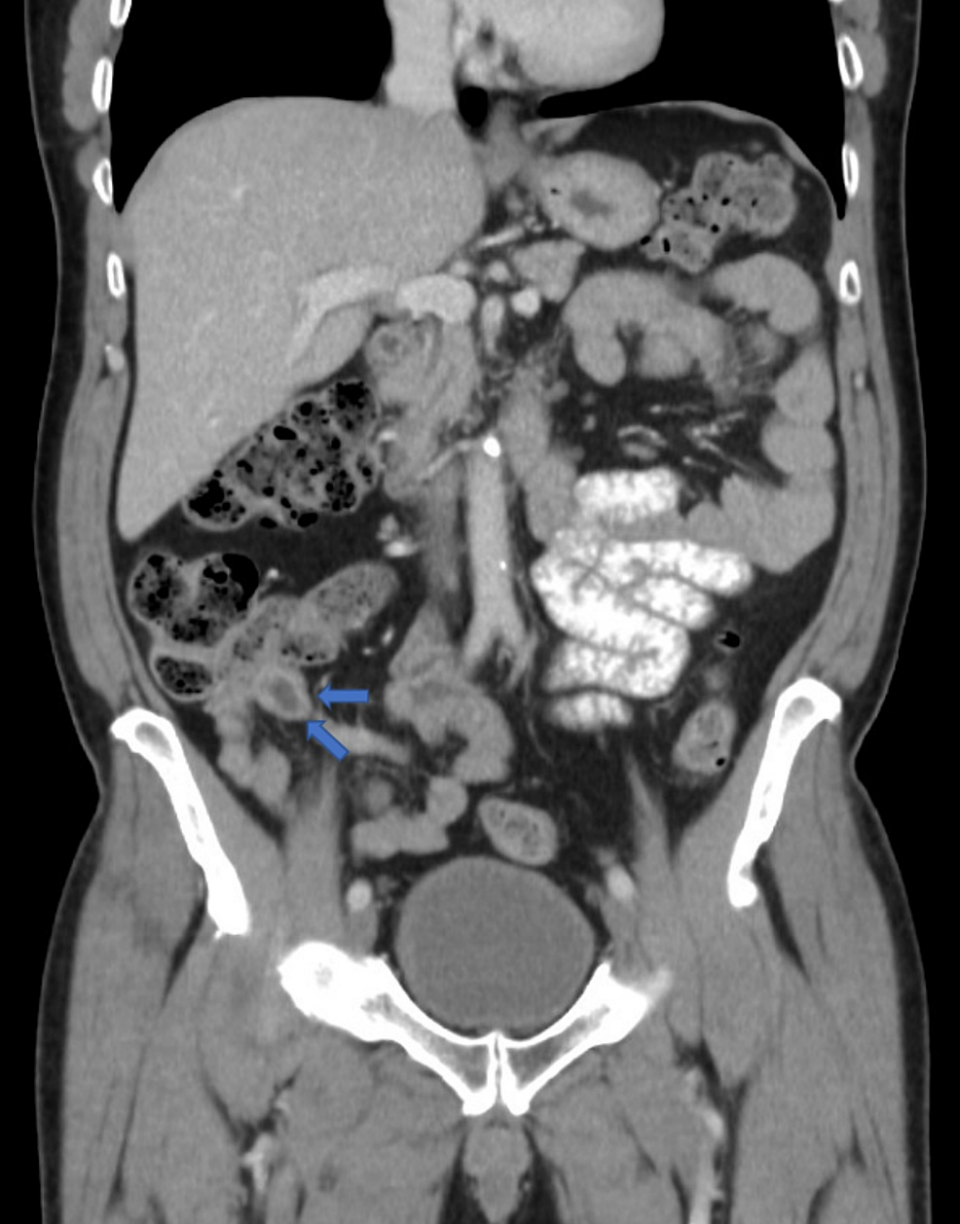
Abdominal coronal view CT scan with contrast demonstrating focal hypodense mass within the base of the appendix, which measures 1cm x 1cm. The more distal portions of the appendix are unremarkable. There is no evidence of periappendiceal fat stranding. CT: Computerized tomography
